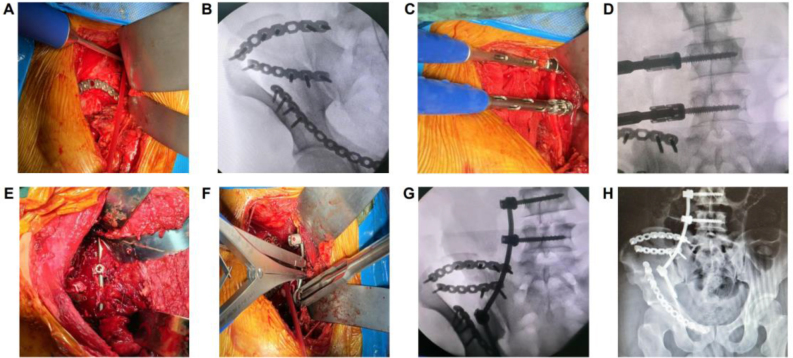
Surgical procedure (A, B). Fixation of ilium and pubis (C, D). Placement of lumbar–iliac screw (E–G). Titanium rod placement and fracture reduction (H). X-ray results of the patient’s pelvis after surgery.
